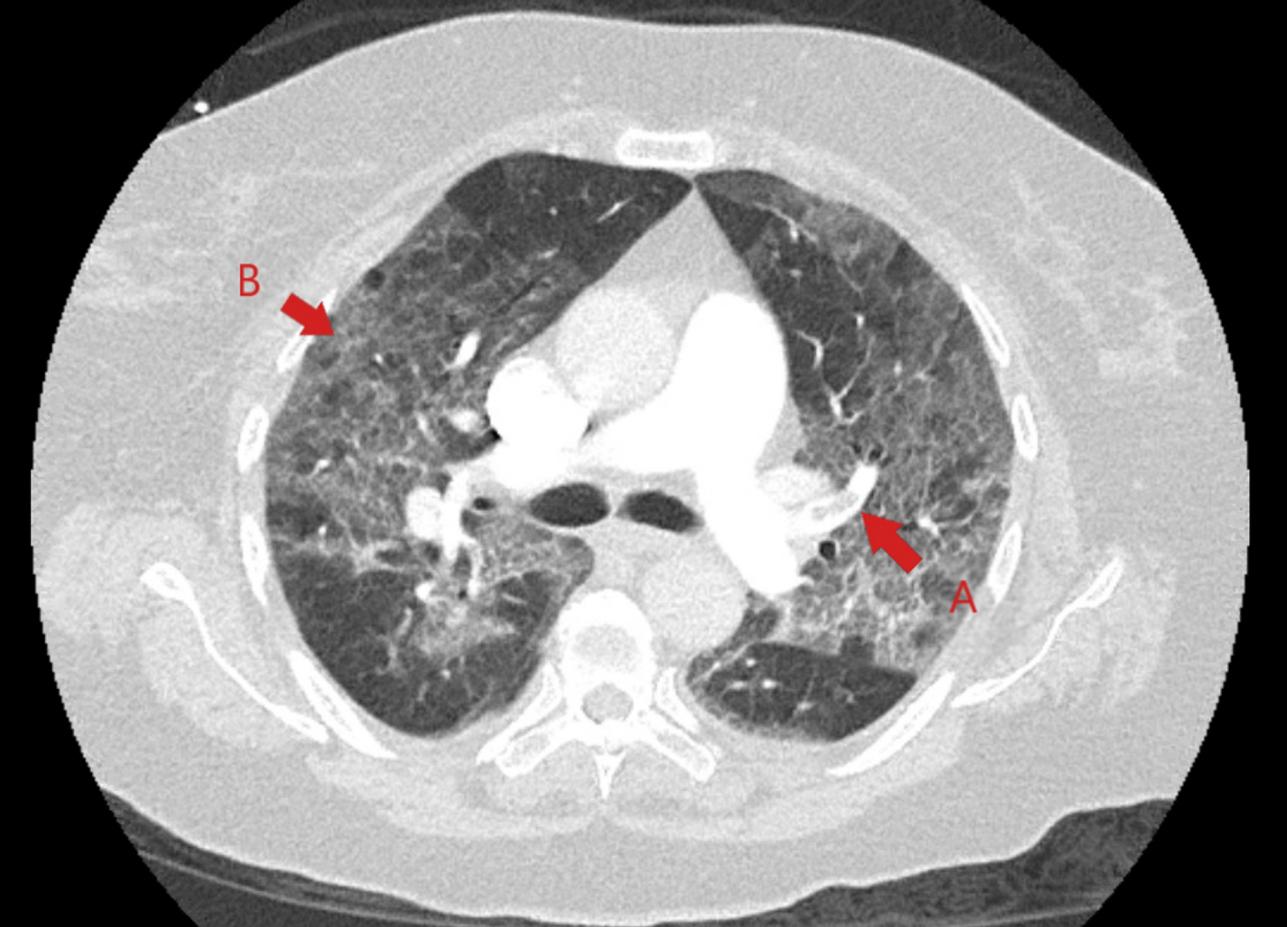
CT chest with IV contrast showed bilateral acute pulmonary thromboemboli (red arrow A) and diffuse bilateral multifocal ground glass opacities (red arrow B). CT, computed tomography
